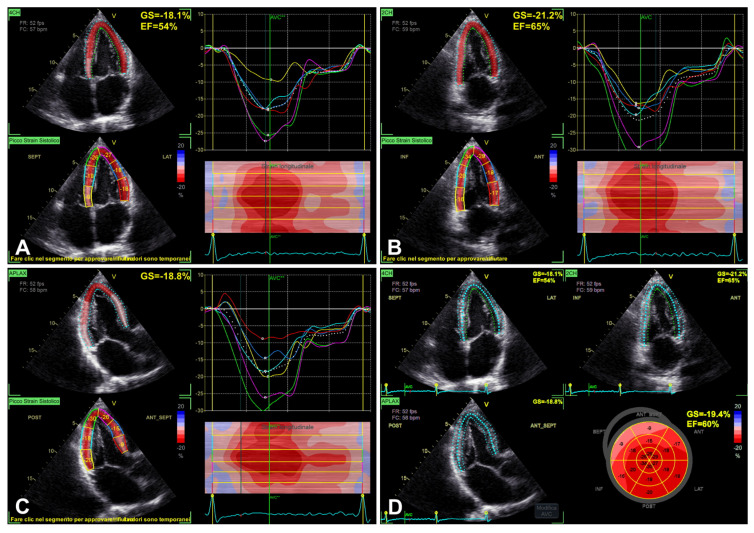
An example of LV GLS evaluation, assessed from 2D apical four-chamber view (A), two-chamber view (B), three-chamber view (C), and reported “bull’s eye” GLS plot (D), respectively. Note: 2D, two-dimensional; GLS, global longitudinal strain; LV, left ventricle/ventricular (see text, tables, and references).
